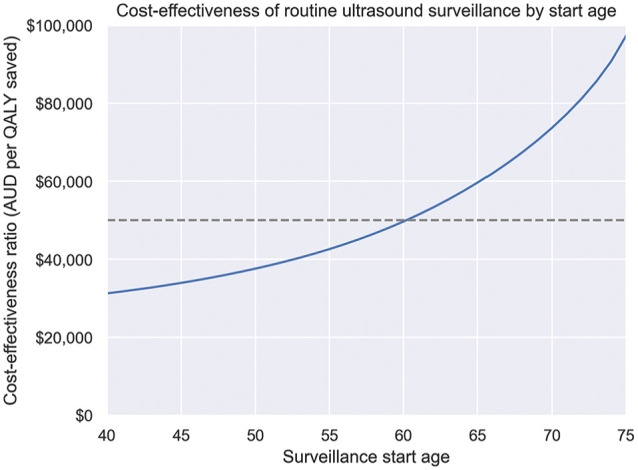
Supplementary analysis: incremental cost-effectiveness ratio for 6-monthly ultrasound surveillance versus no surveillance, by age of cirrhosis diagnosis. The $50,000/QALY willingness-to-pay threshold is indicated. QALY, quality-adjusted life-year; AUD, Australian dollars.
